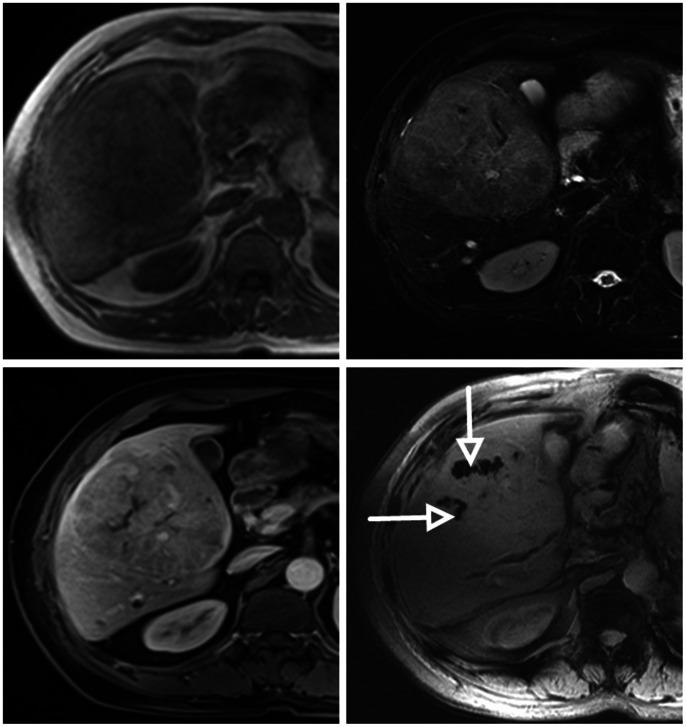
A case of a 72-year-old man with HCC. a, b, and c correspond to T1 precontrast, the axial T2-weighted imaging, and T1-weighted DCE, respectively; d: axial non-contrast SWI indicates intratumorally linear vasculatures or curved cylindrical tube-shaped structures with clear boundaries (arrows).
